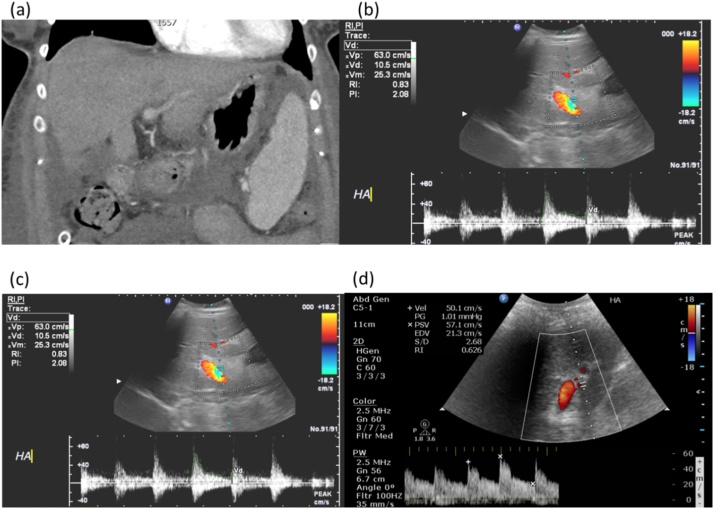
Post-operative imaging exams. Computerized tomography at post-operative day 11 showed the site of arterial anastomosis (white arrow) naturally aligned without redundancy (*panel a*). Ultrasonography performed for case1 (*panel b*), case 2 (*panel c*) and case 3 (*panel d*) at post-operative day 11 showed resistance index of 0.83, 0.54 and 0.63, respectively. For all cases the arterial flow was normal without evidence of hepatic artery thrombosis.
